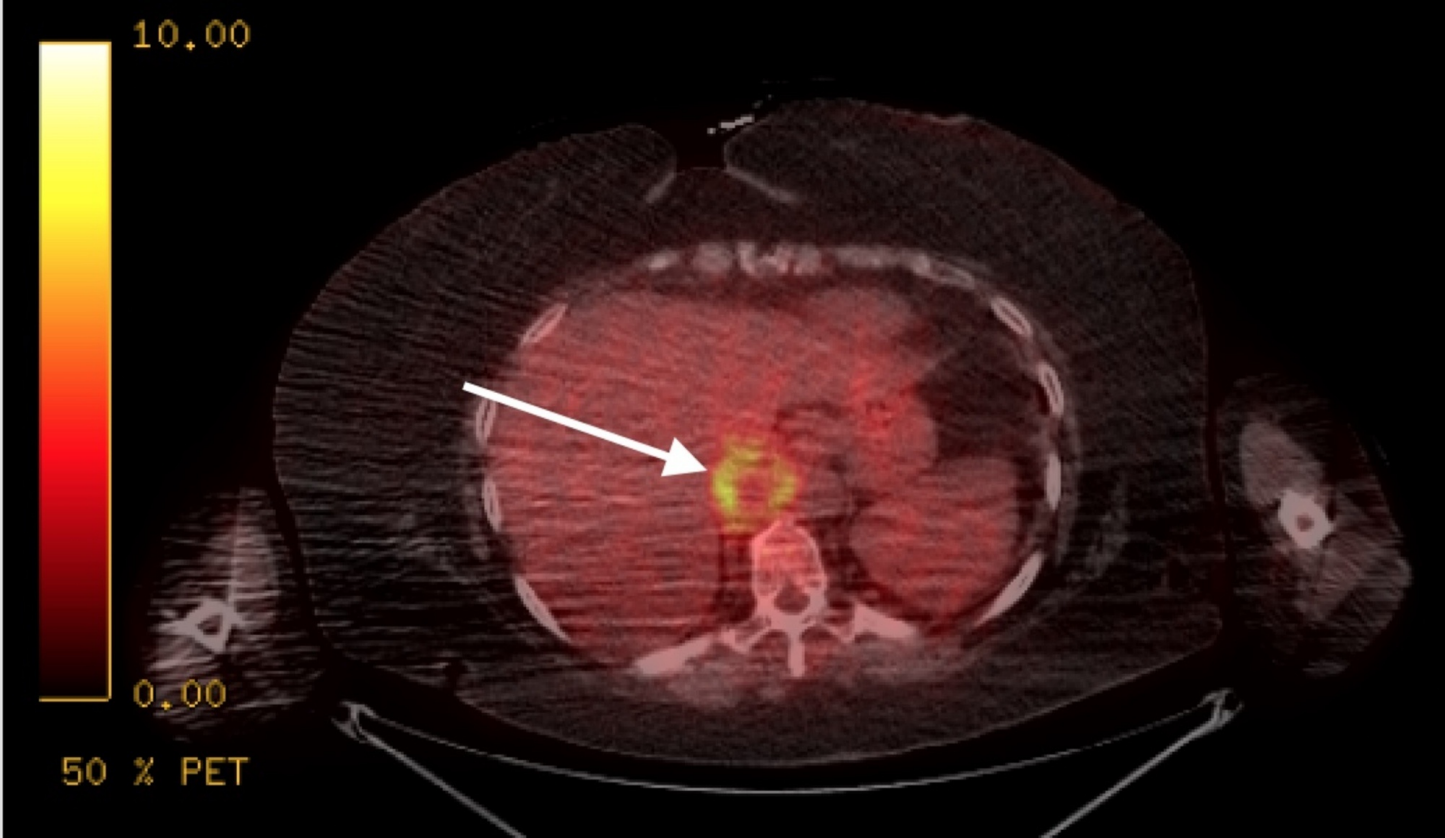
Positron emission tomography-computed tomography (PET-CT) scan of the patient showing a hypermetabolic, enlarged periaortic lymph node to the right of the aorta at the level of the diaphragm (see arrow).
